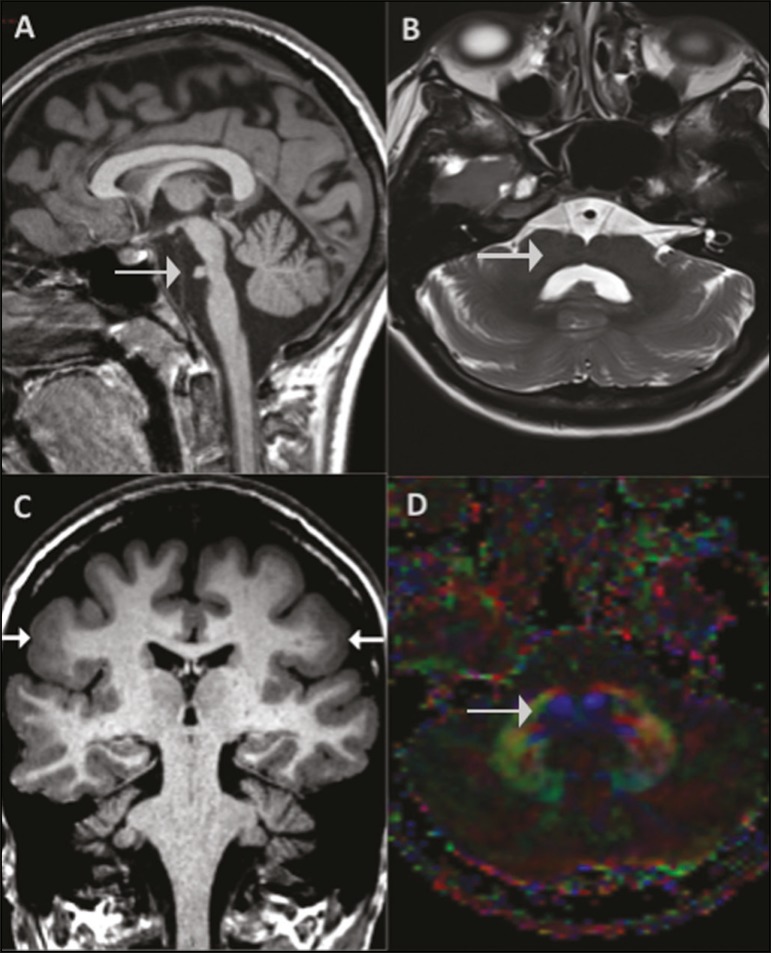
A: Sagittal T1-weighted image depicting a short pons (arrow).
